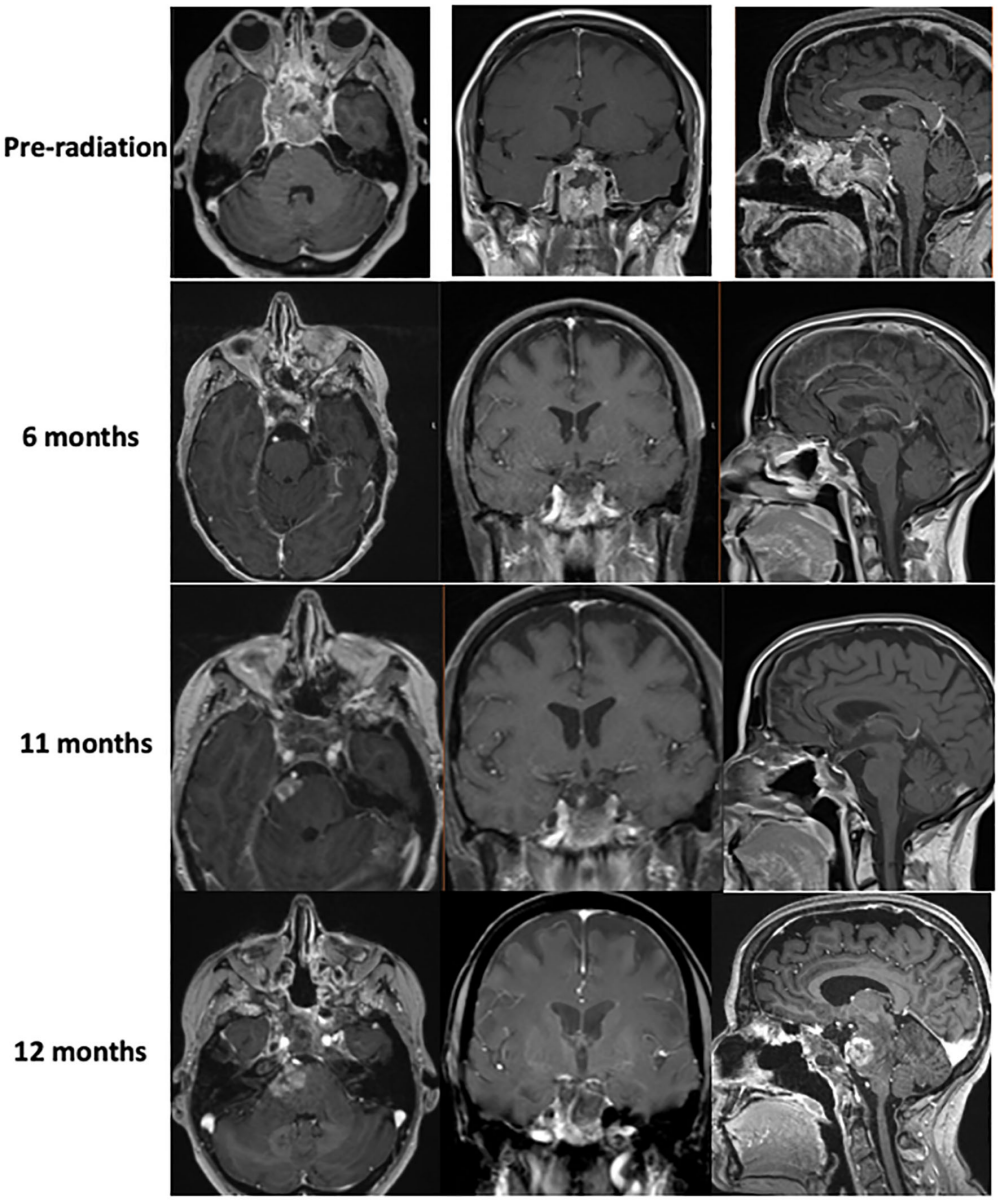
MR imaging showing tumor hyperprogression prior to radiation therapy, as well as tumor response to chemotherapy 6 and 11 months out from diagnosis. Imaging shows evidence of brainstem invasion at 12 months.
